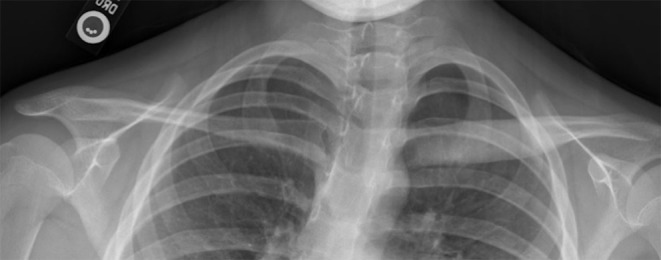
Involvement of the left clavicle. Radiograph obtained 2 years after disease onset showing that the left clavicle is broader and denser than the right, indicating chronic changes.
